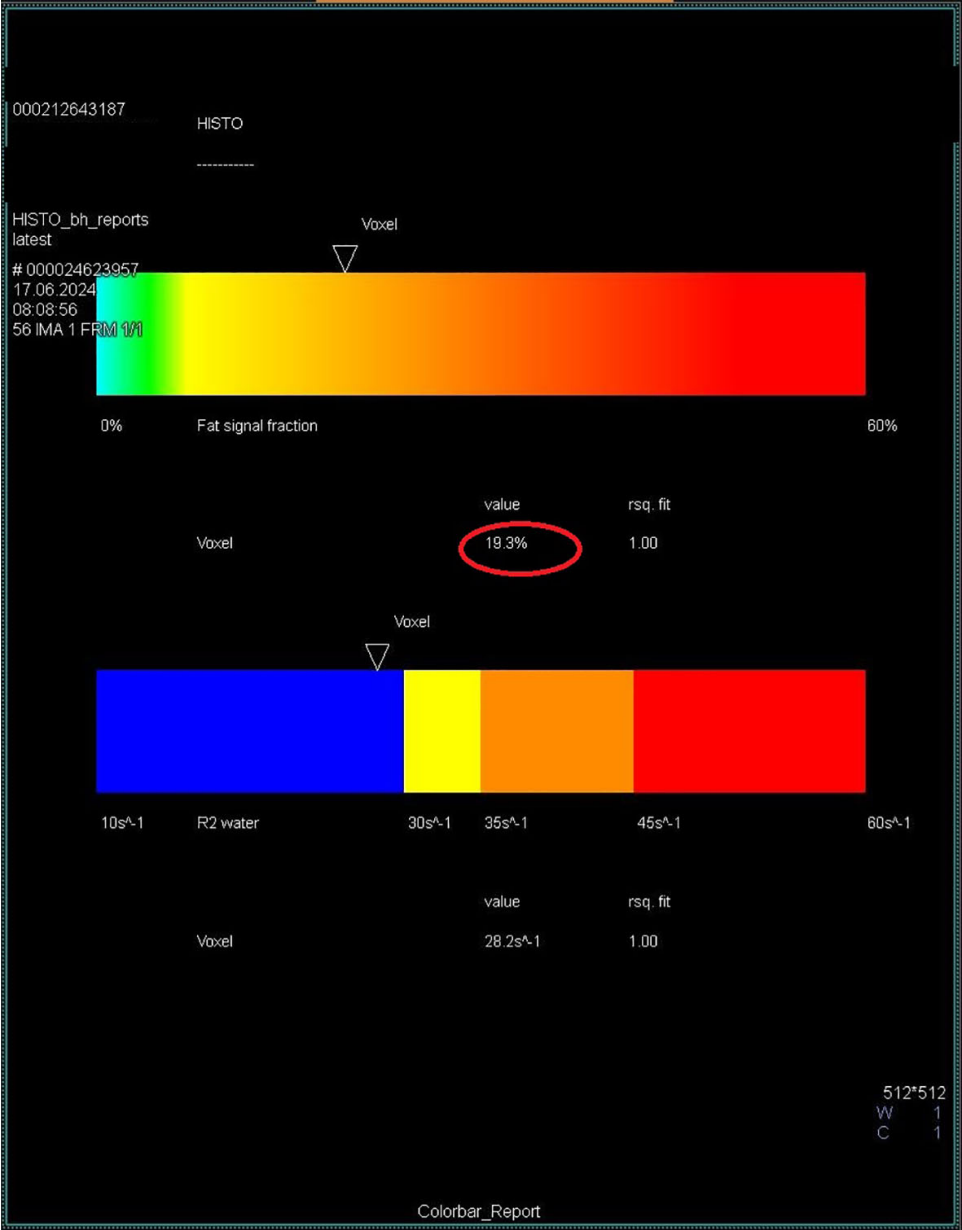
Case 2- Liver MRI image 2 (19.3% of fat content, N<5%).
